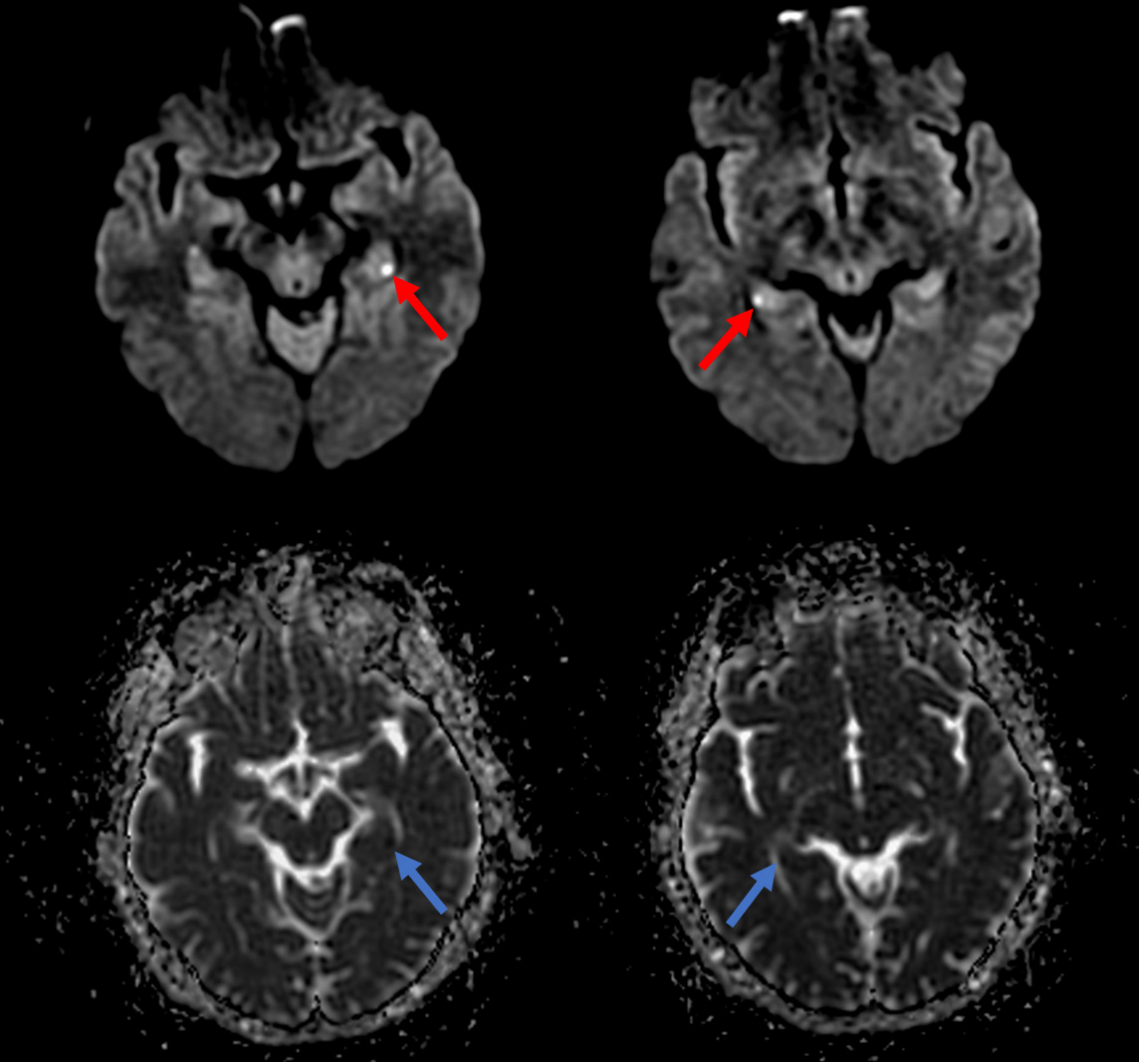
Selected axial diffusion-weighted imaging (*b* = 1000, 4 mm slice thickness) MR images (above) and corresponding apparent diffusion coefficient (ADC) maps (below) demonstrating bilateral punctate hyperintensities in the CA1 region of the hippocampi (red arrows) with corresponding signal loss on ADC (blue arrows).
